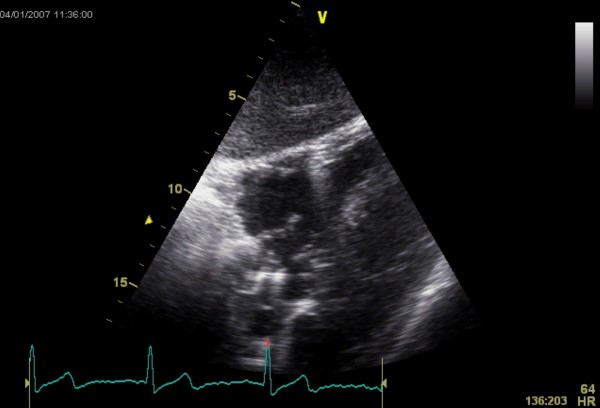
Transthoracic echocardiogram showing a large right atrial vegetation.
